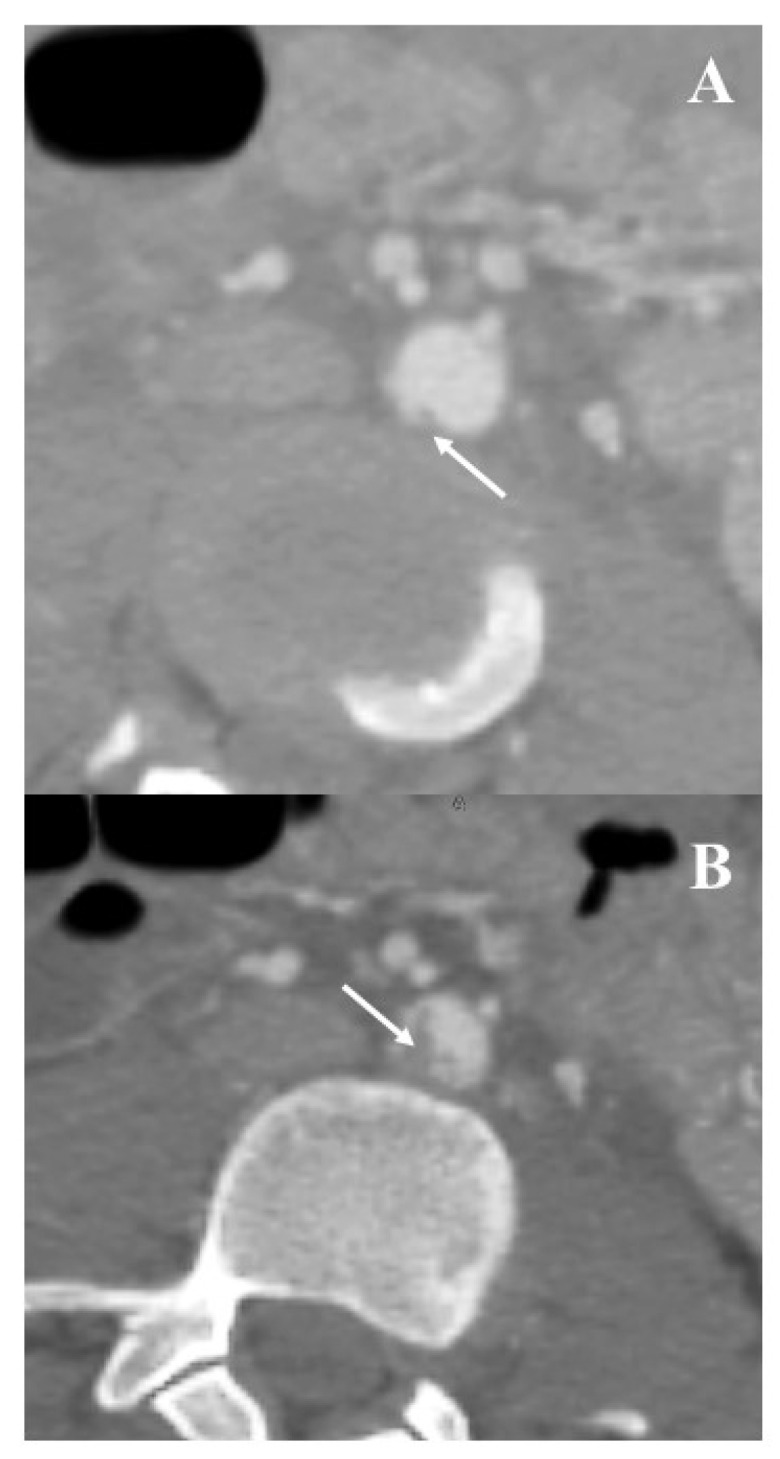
Patient #3: preoperative computed tomography-angiography showing the disruption of the intimal contour ((A), white arrow) with a large intimal flap ((B), white arrow).
